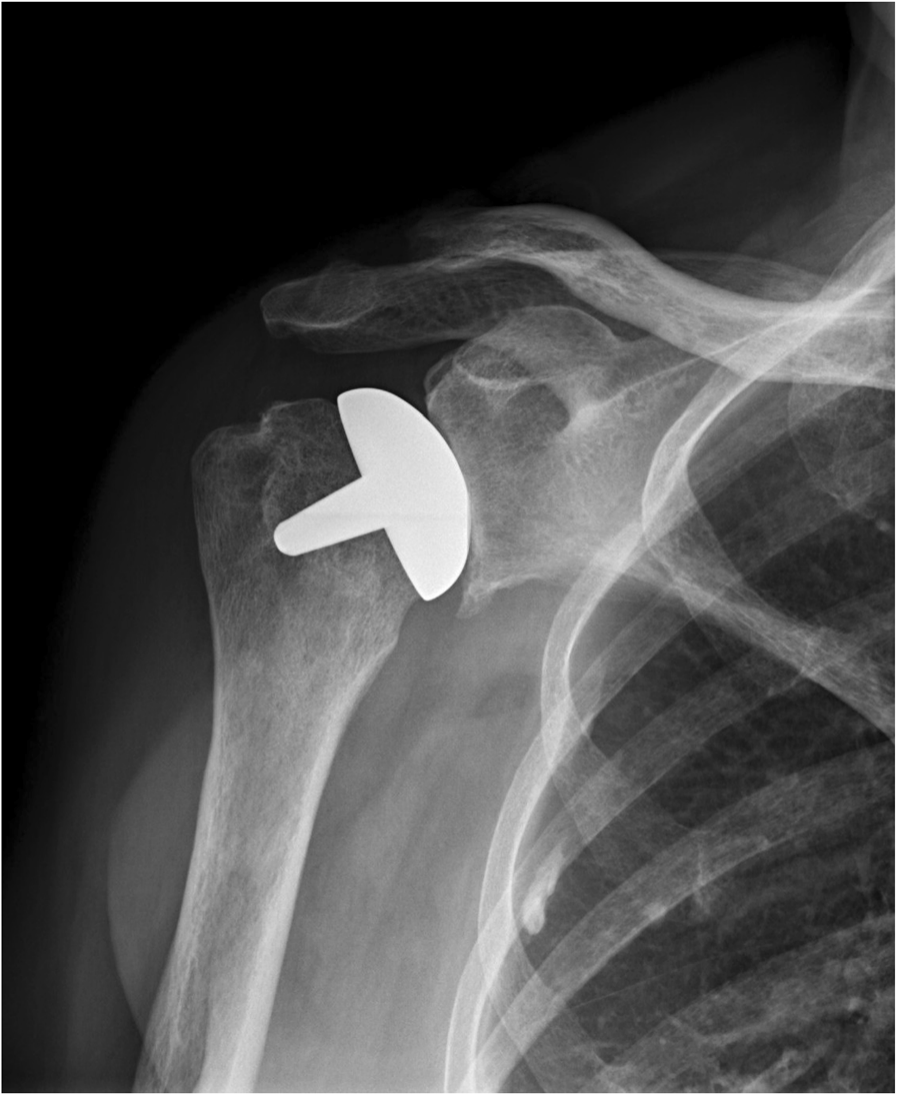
Post-implant AP radiograph where all observers classified the size of the implant as too small
